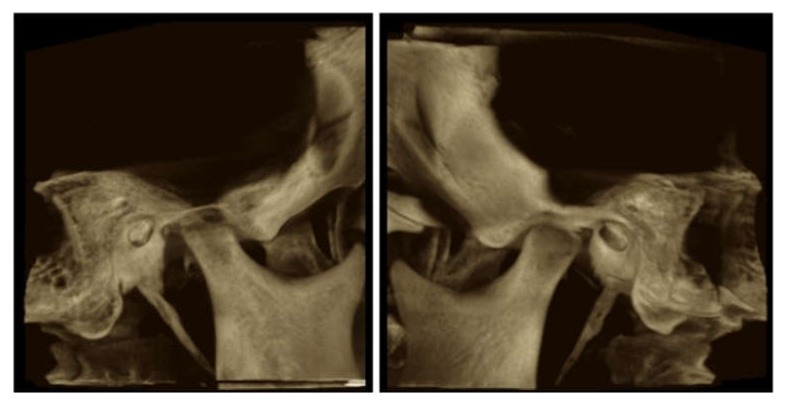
CBCT-condylar lysis and condylar remodeling.
